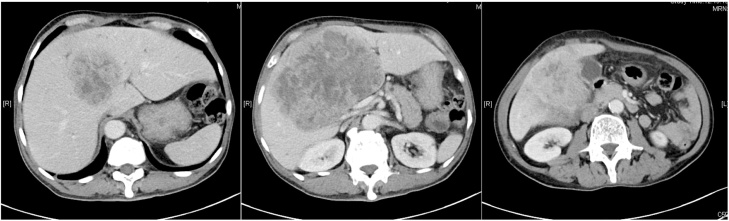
Axial image of CT scan before Y90 therapy: an HCC lesion 15 cm in size.
